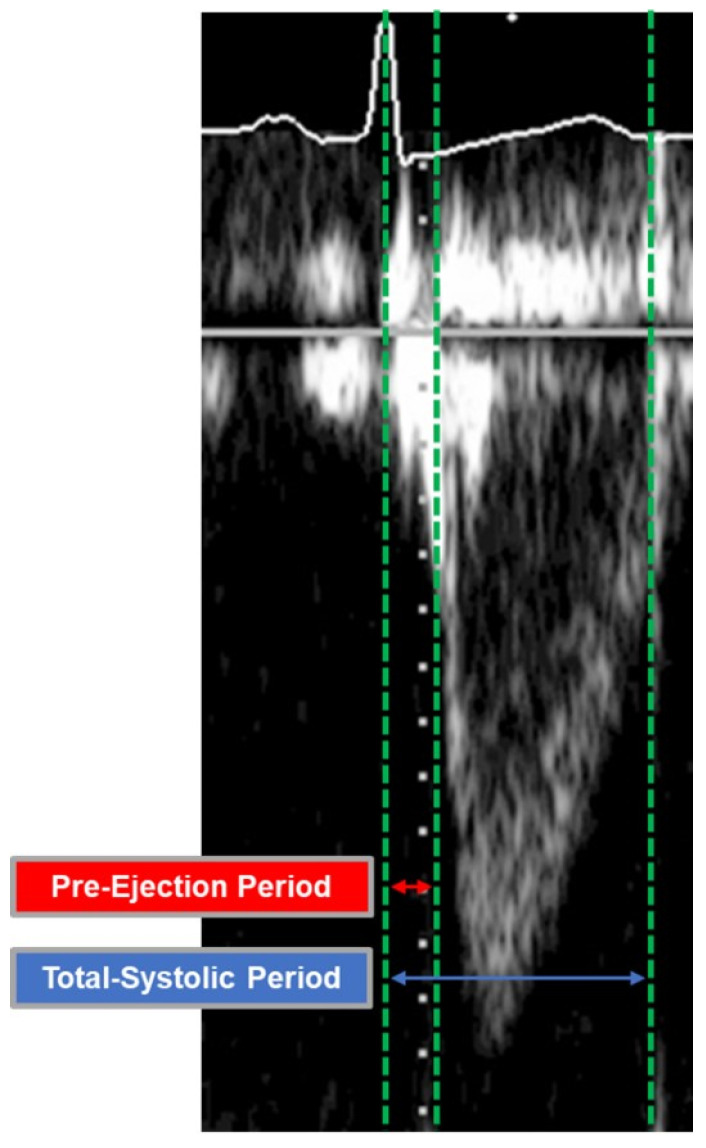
Assessment of the Pre-Ejection and the Total-Systolic Period of the Left Ventricular Outflow Tract. A pulsed wave Doppler was positioned over the aortic valve in apical five-chamber view.
